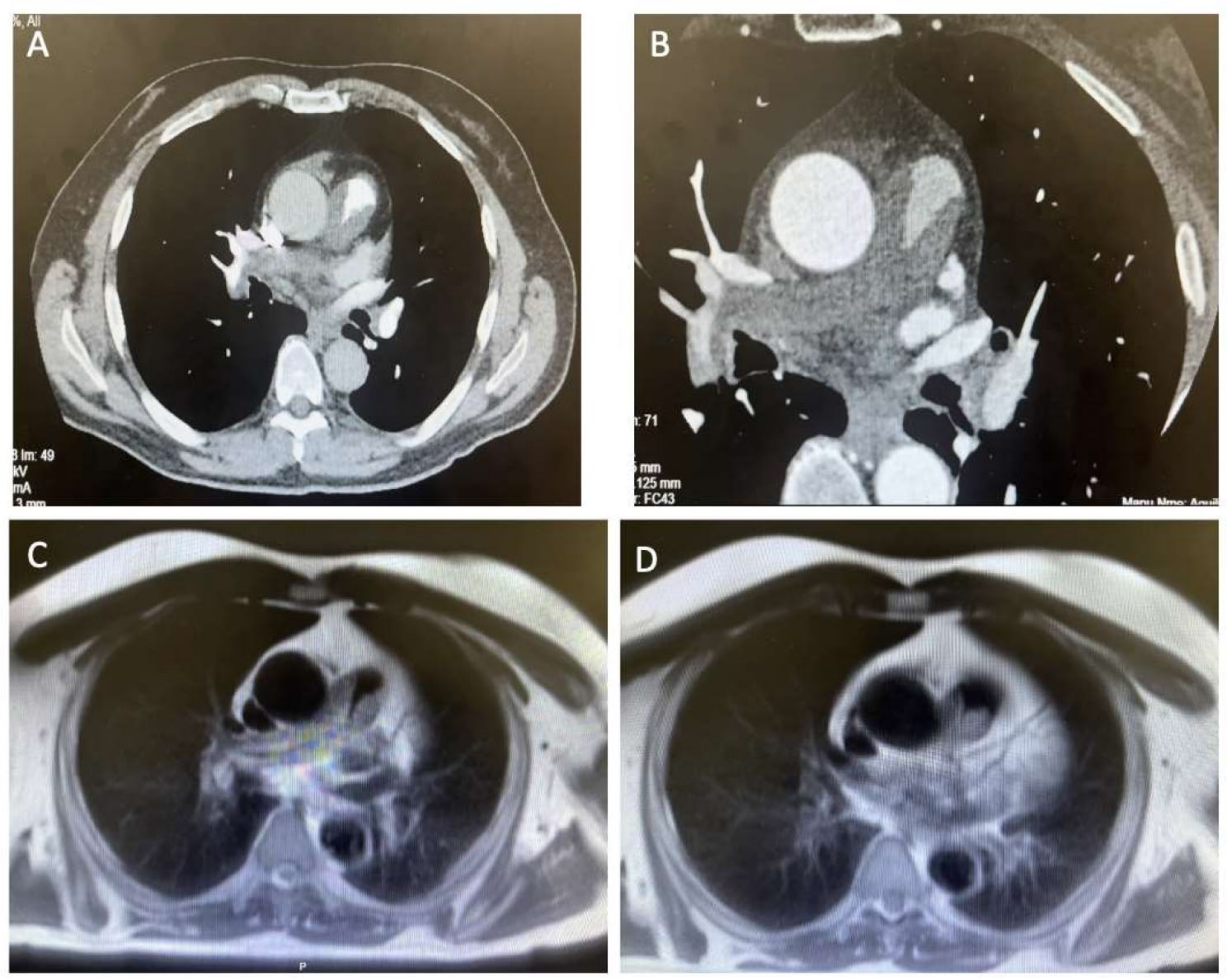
Images A and B show pre-operative CTPA and images C and D show pre-operative cardiac MRI
